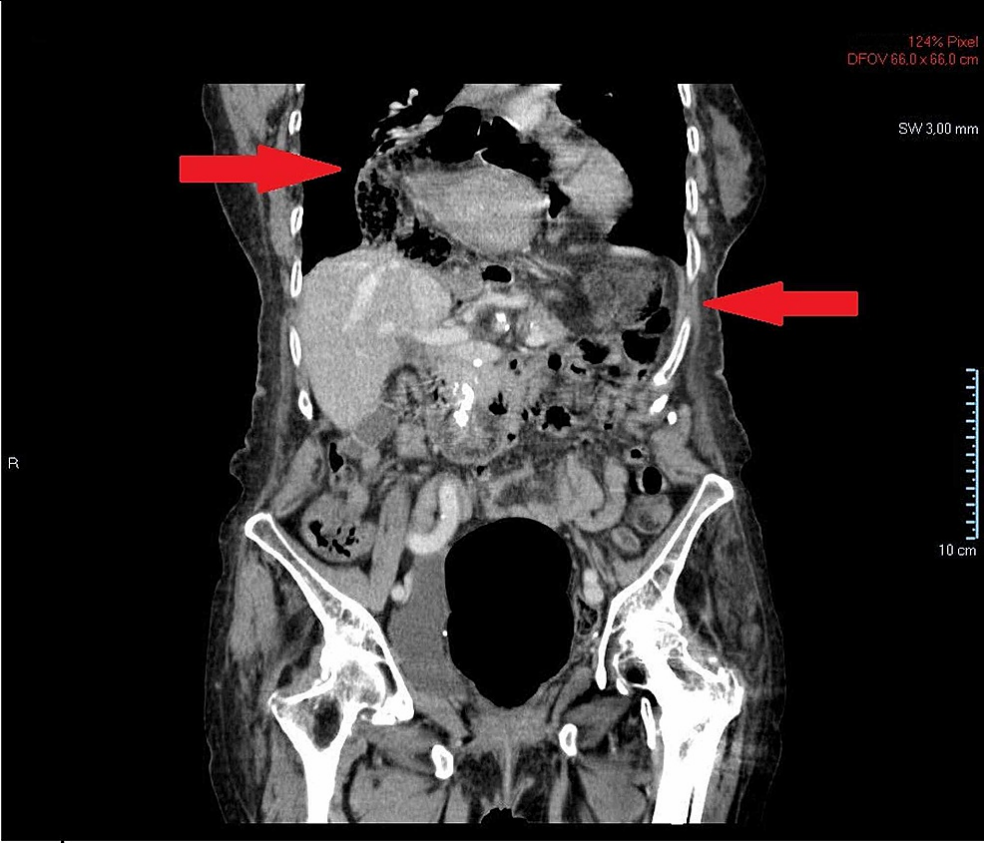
Coronal segment of a CT scan showing a distended stomach, with a major portion in the intrathoracic segment. The right arrow shows a stomach portion within the thoracic cavity and the left arrow shows another stomach portion within the abdominal cavity.
